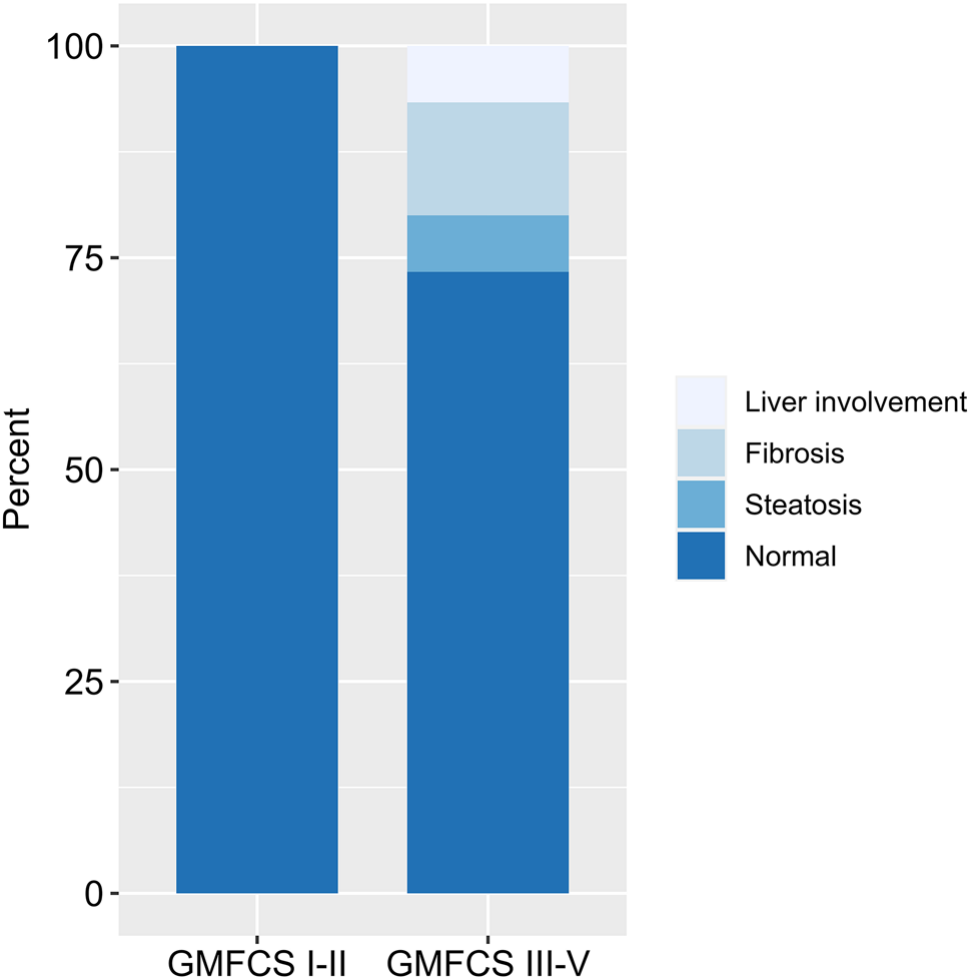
Percentage of liver fibrosis and/or steatosis assessed by ultrasound and FibroScan in children with cerebral palsy, grouped according to gross motor function. Abbreviations: Liver involvement = steatosis and fibrosis, GMFCS = gross motor function classification system
